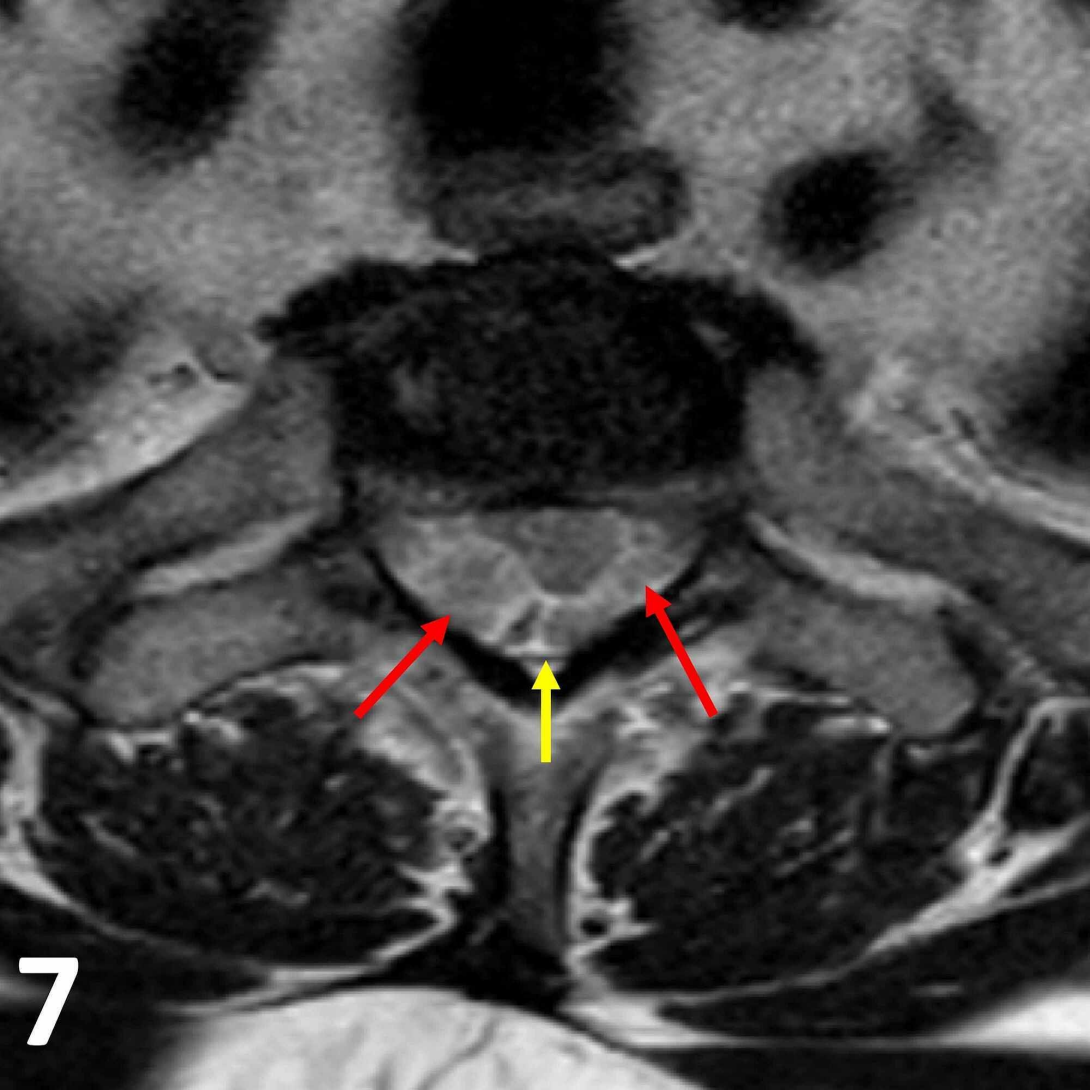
Axial T2 MRI image at the T1/T2 vertebral level. Note the T2 heterogeneously hyperintense intradural lesions (red arrows) within the thecal sac (yellow arrow). The two lesions, particularly the larger right-sided one, are indenting the spinal cord without causing spinal cord compression.
